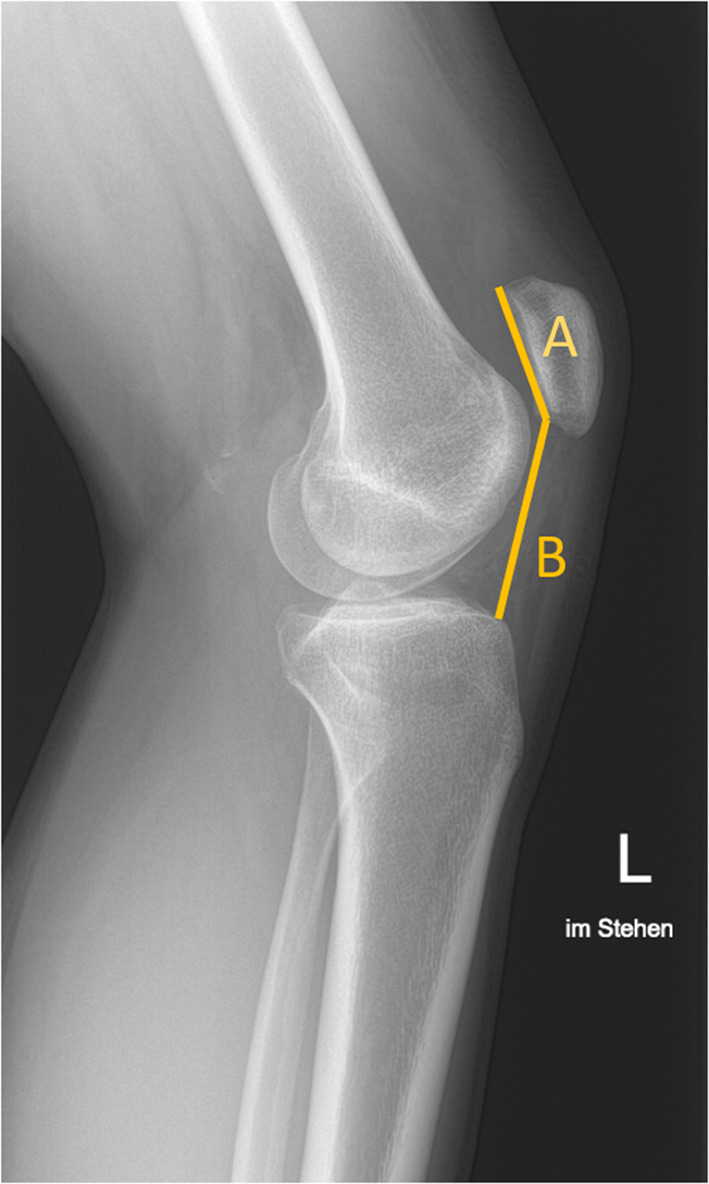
Conventional X-Ray lateral view. Cato–Deschamps (CD) index measurement. A patellar length, B distance from the patella to the tibia. CD: B/A
